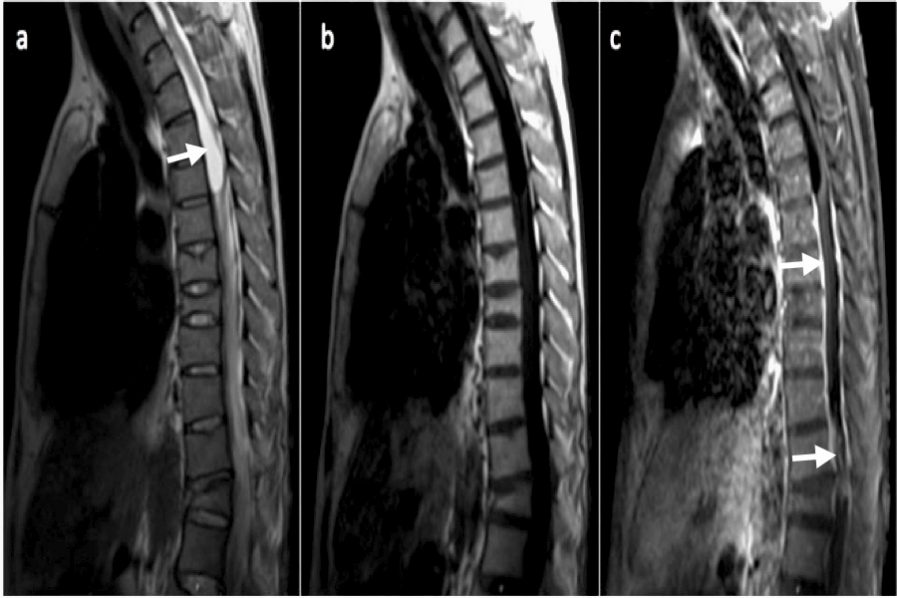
MRI of thoracic spine with and without contrast [a] Sagittal T2W shows an intradural and partially intramedullary elongated cystic structure (arrow) in the upper thoracic spine with marked distortion and compression of the spinal cord. Also, the spinal cord with abnormal T2 hyperintensity is seen with septated and tethered components diffusely. [b] T1W C- and [c] T1W C+ show an epidural and intradural enhancement with intramedullary irregular areas of enhancement in the distal cord (arrows). MRI: magnetic resonance imaging
